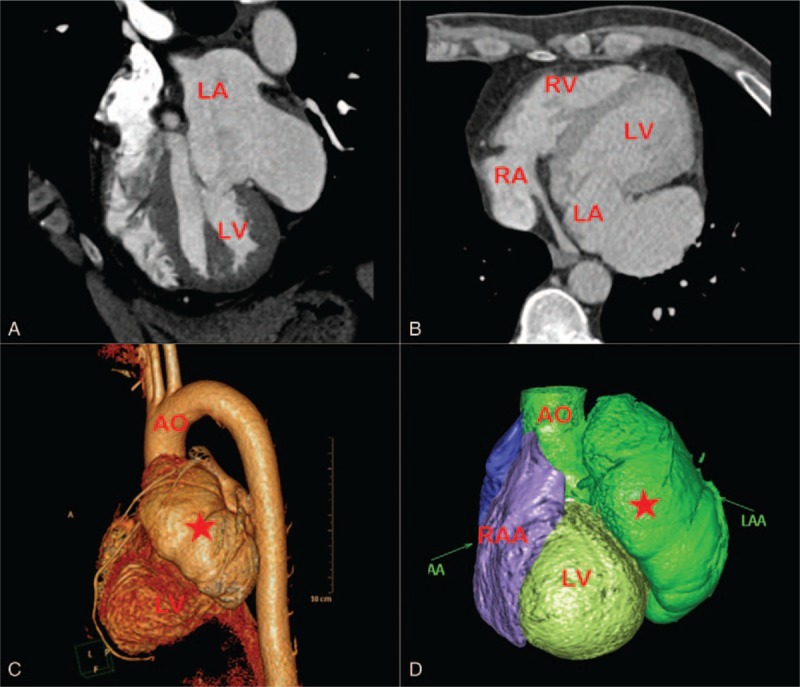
CT views characterizing the giant LAA measuring 9.6 × 4.5 × 3.8 cm. AAO = ascending aorta, LA = left atrium, LAA = left atrial appendage, LV = left ventricle, RA = right atrium, RV = right ventricle, RAA = right atrial appendage.
